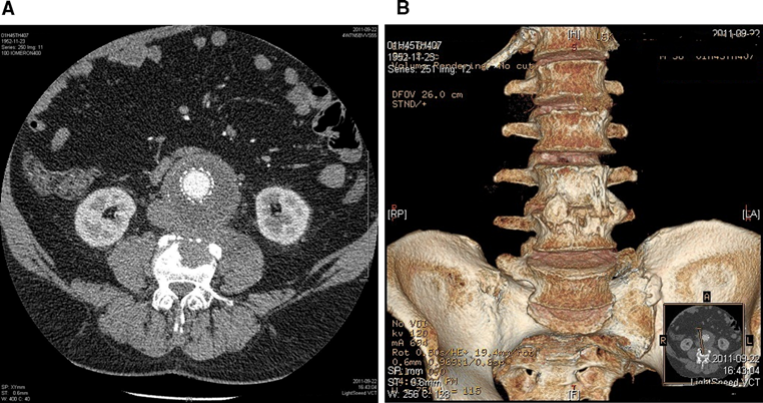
Angio-CT axial scan of aneurysm below renal arteries after EVAR. A Regression of the inflammatory changes in the vicinity of the aorta and iliopsoas muscle is evident. Visible bone remodeling and new bone formation of damaged L4 body are present. B 3-D reconstruction of the lumbar spine reveals a proliferative reaction at the level of the affected vertebrae
